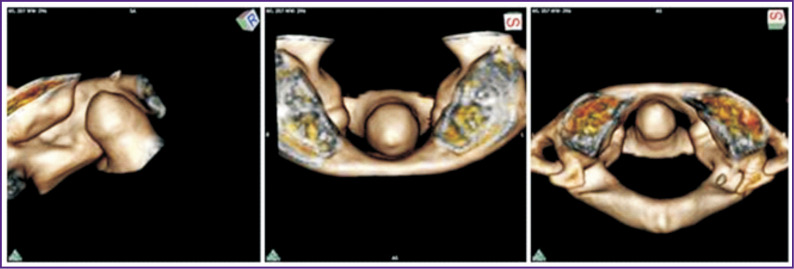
3D reconstruction of CT image of median atlantoaxial joint for an 18-year old man
